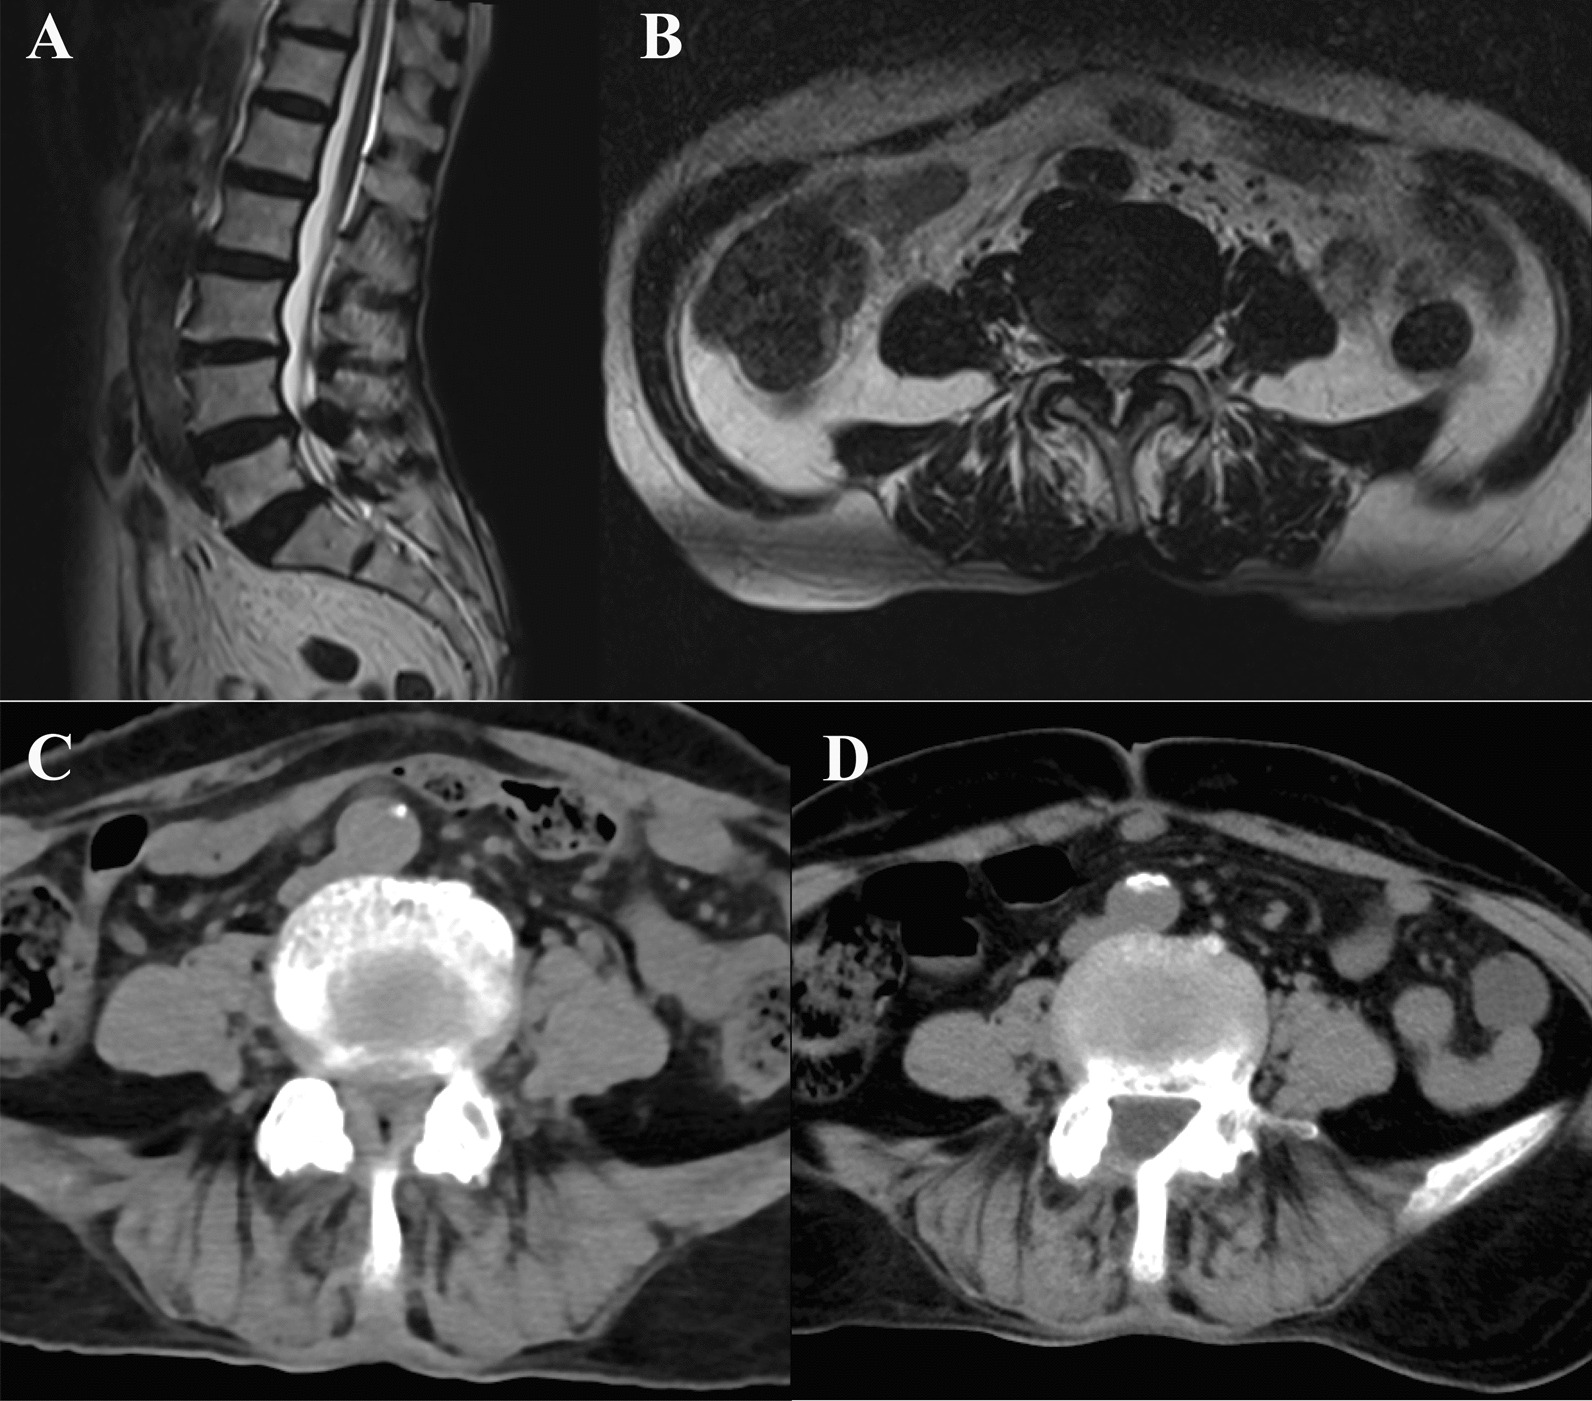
Imaging data of a typical case of L4/L5 lumbar spinal stenosis undergoing AUSS treatment. A, B Preoperative magnetic resonance imaging. C Preoperative computed tomography. D Computed tomography 1 week after surgery
